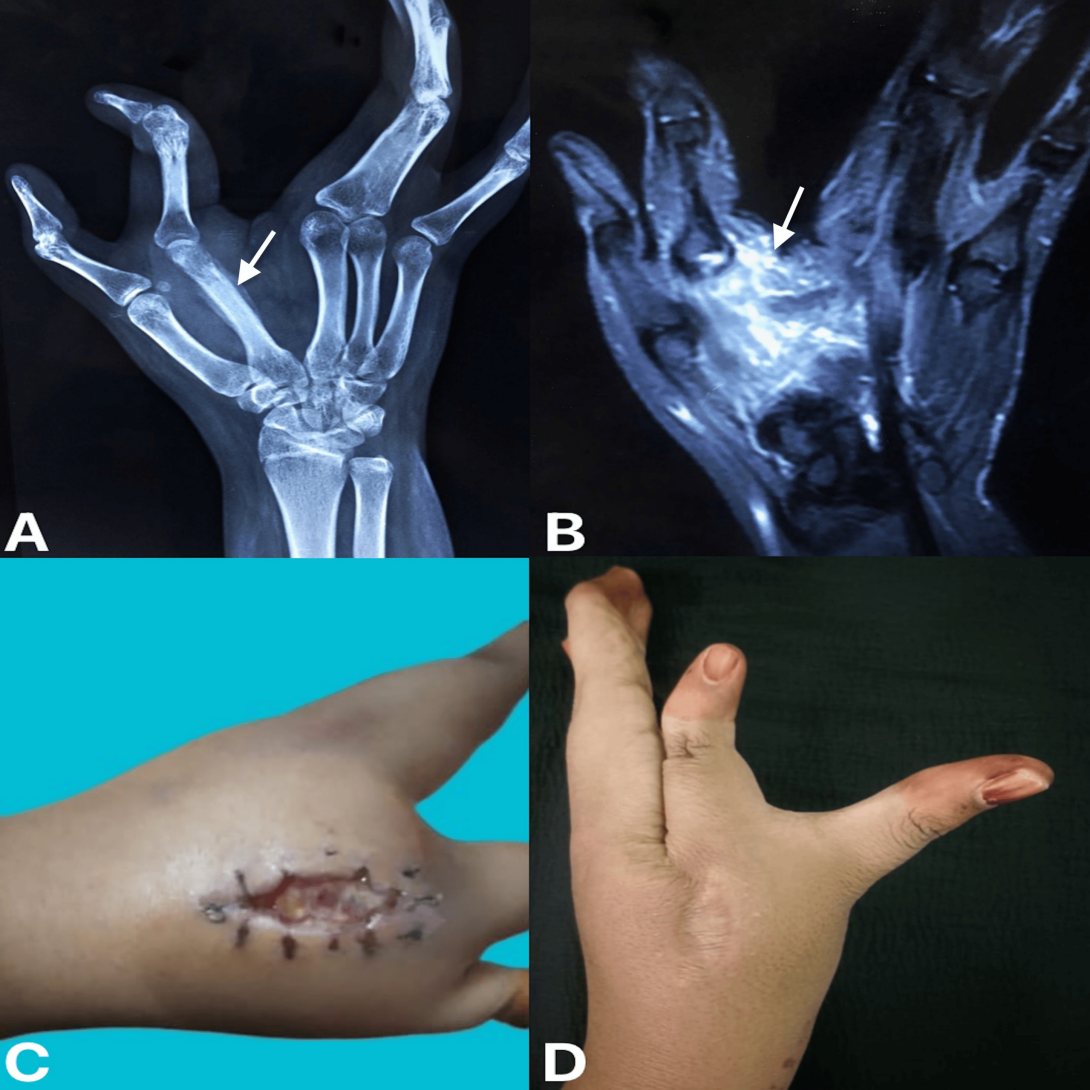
(A) Radiograph of left hand showing the anterior-posterior view, (B) magnetic resonance imaging of left hand showing coronal section, (C) four weeks post-operative wound showing sinus over the second metacarpal, and (D) 1.5 years post-operative clinical picture of left hand (A) Arrow shows rarefaction of the second metacarpal bone. (B) Arrow shows edema with fluid collection in the region of the second metacarpal bone.
